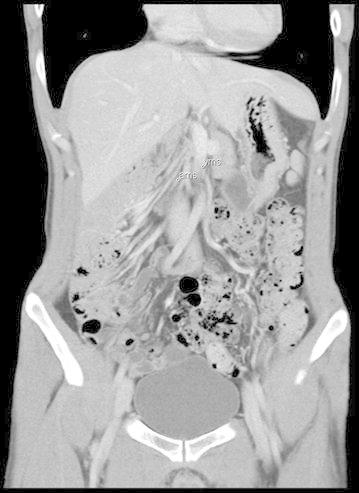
CT scan showing inverted vessels, front view
